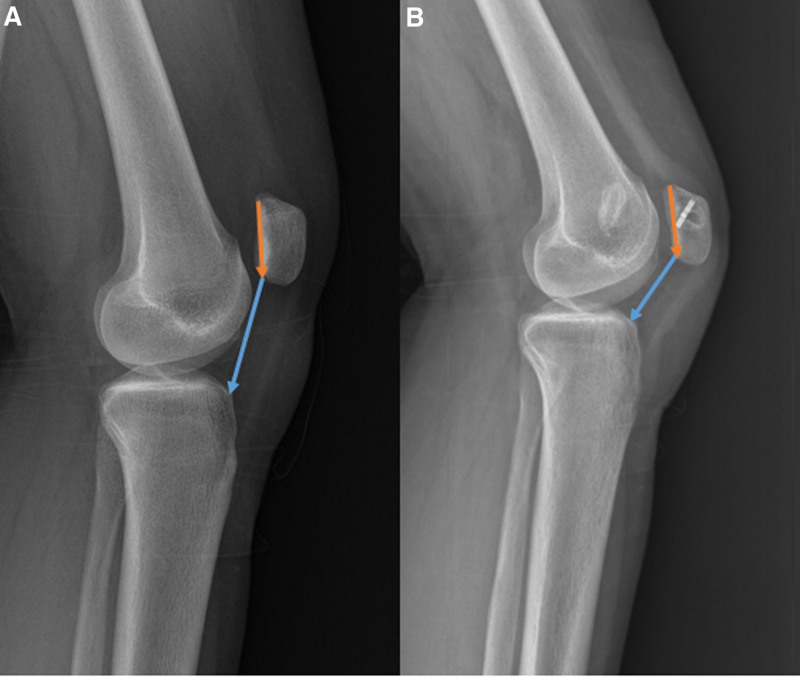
Preoperative (A) and postoperative (B) lateral radiographs of the knee at 30° flexion. The radiographs show patellar height indices. Caton-Deschamps index ratio of the distance between the anterosuperior point of the tibial plateau and distal pole of the patella (blue line) to the joint patellar surface (orange line).
